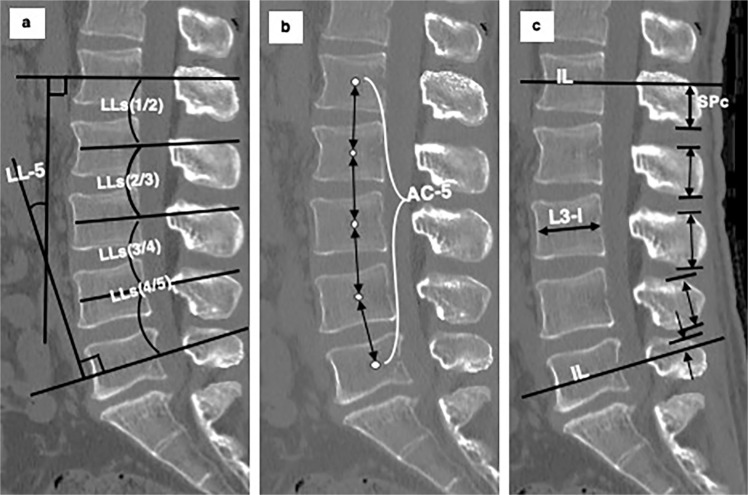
Definitions of L1-L5 parameters in mid-sagittal computed tomography images. (a) Lumbar lordosis (LL-5): the angle between intersecting lines drawn through the centres of the anterior and posterior walls of the vertebral bodies (IL) of L1 and L5. LL-5 is the sum of segmental lordosis (LLs) from L1/2 through L4/5. (b) Sum of the anterior spinal column height (ASC-5): the sum of the distances between the centres (white dots) of the adjacent vertebral bodies of L1-L2 through L4-L5. (c) Sum of spinous process heights (SP-5): the L1 SPc height (the height of the caudal part of the spinous process) + whole SP heights of L2, L3 and L4+ L5 SPr height (the height of the rostral part of the spinous process).
